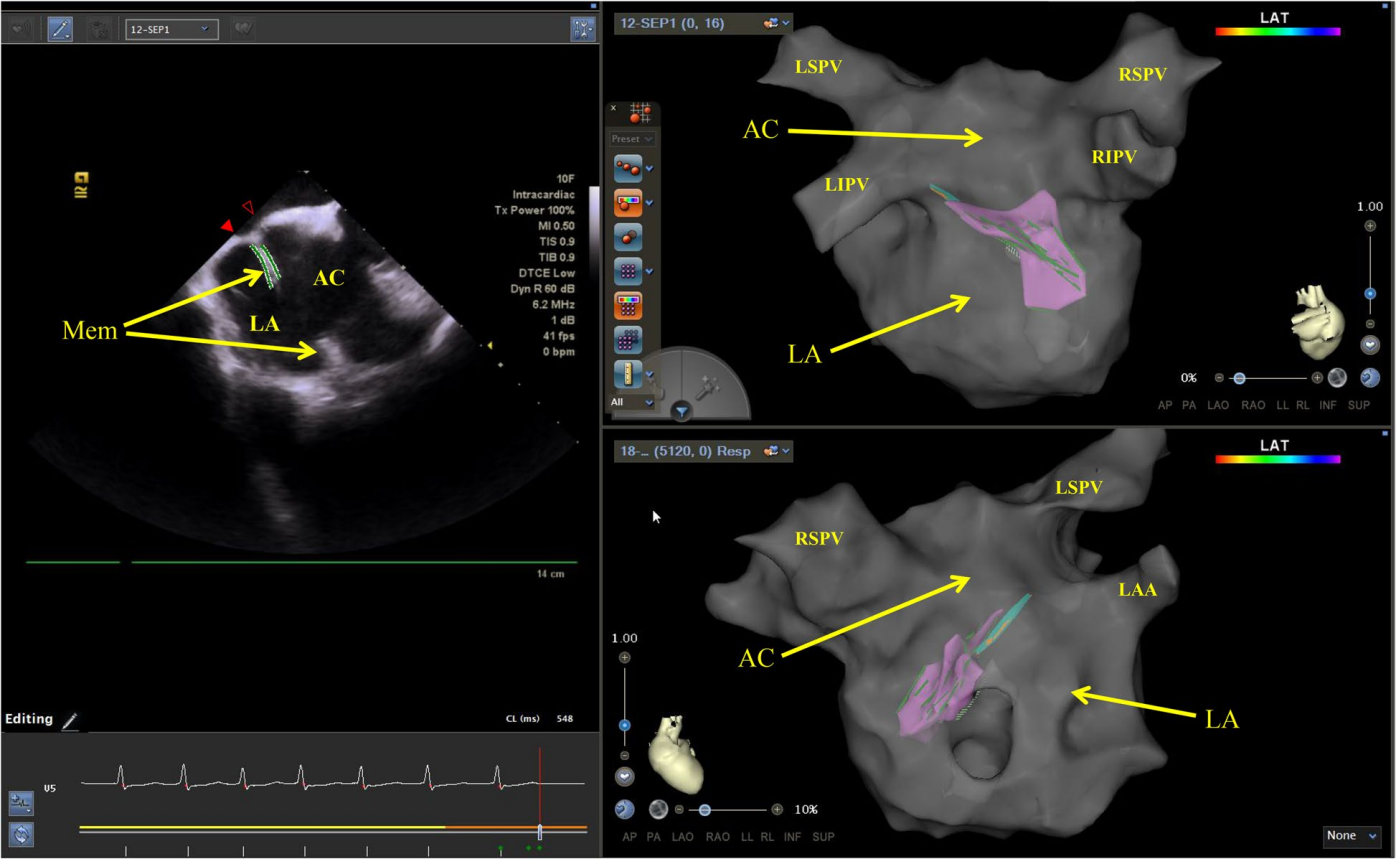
Three-dimensional intracardiac ultrasound modeling image. The pink color indicates the membrane, red hollow triangles indicate the septum entering the accessory chamber, and red solid triangles indicate the septum entering the true left atrial chamber. Mem: membrane; AC: accessory chamber; LA: true left atrial chamber; LAA: left atrial appendage; LSPV: left superior pulmonary vein; LIPV: left inferior pulmonary vein; RSPV: right superior pulmonary vein; RIPV: right superior pulmonary vein
